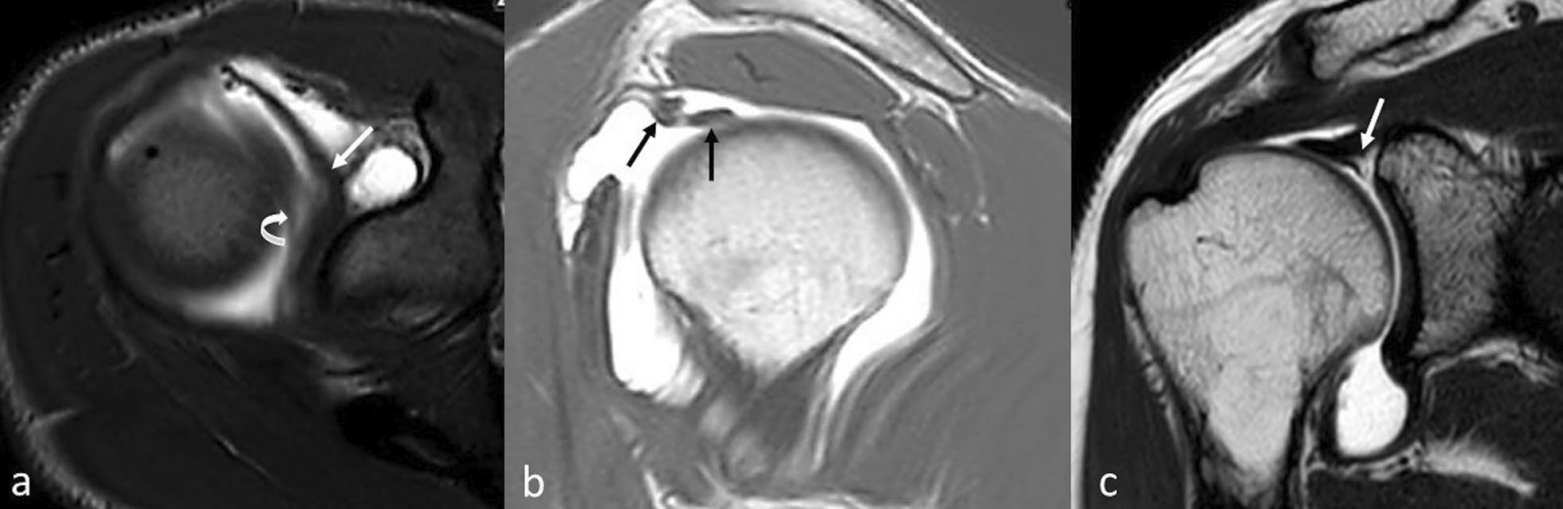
a MR arthrography, T1-weighted with fat signal saturation (FAT-SAT) imaging on axial plane. SPL-DO anatomical variant (long head biceps tendon has double band) comes from supraspinatus tendon (white curved arrow) and from glenoid (white arrow). b MR arthrography, sagittal SE T1-weighted. SPL-DO anatomical variant (double beams of long head biceps tendon; black arrows). c MR-arthrography, coronal SE T1-weighted. SPL-DO anatomical variant is associated with SLAP lesion type IV (white arrow)
